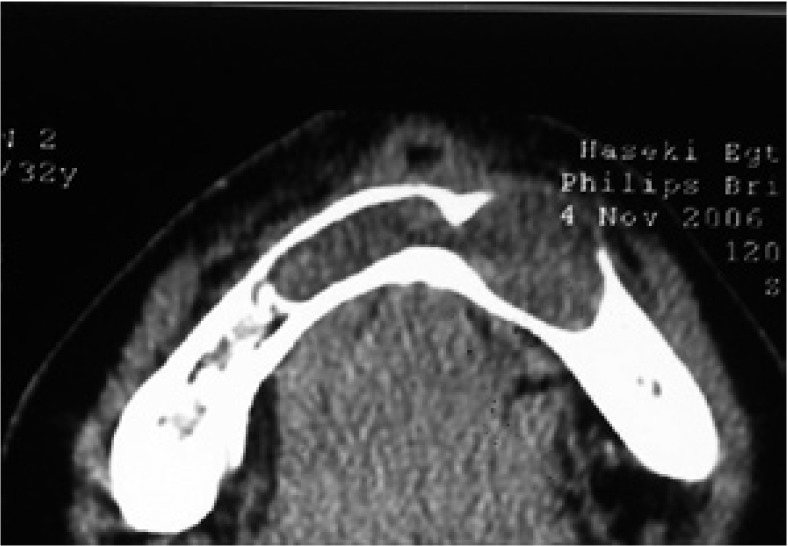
CBCT shows a large KCOT with soft tissue algorithm in the right mandibular symphysis and cortical expansion. Note scalloped border.
